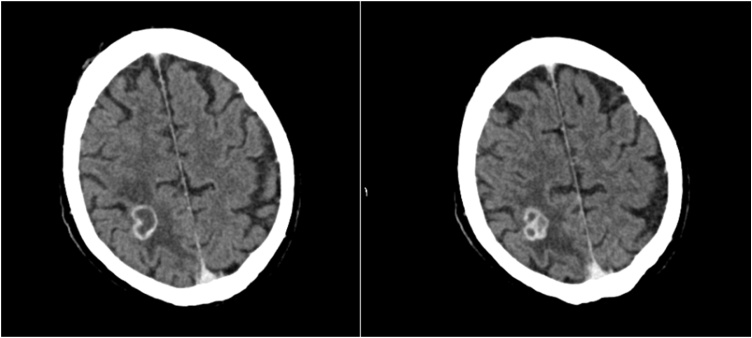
CT Scan with contrast with a 19 × 18 × 20 mm lesion and minimal regression of the surrounding edema.
